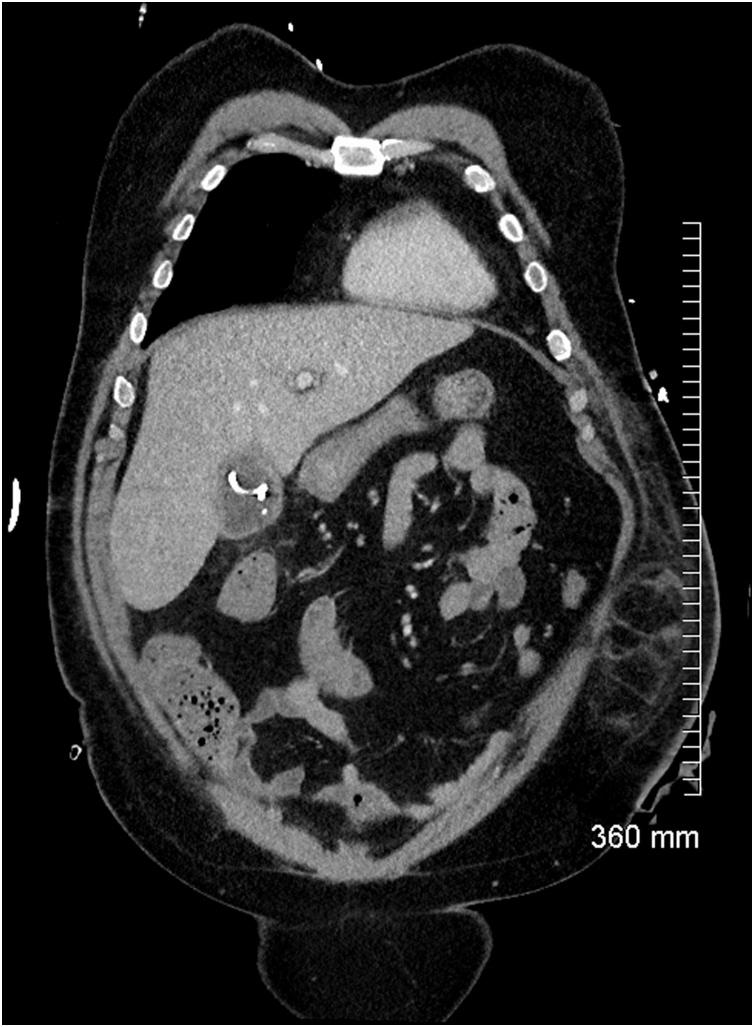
Coronal CT showing interval improvement in gallbladder distension and pericholecystic inflammatory changes with a cholecystostomy drain in situ.
